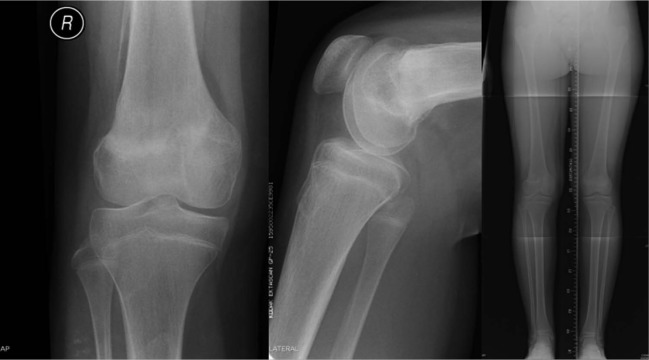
Radiographs at 1 year demonstrating satisfactory reduction and leg length.
